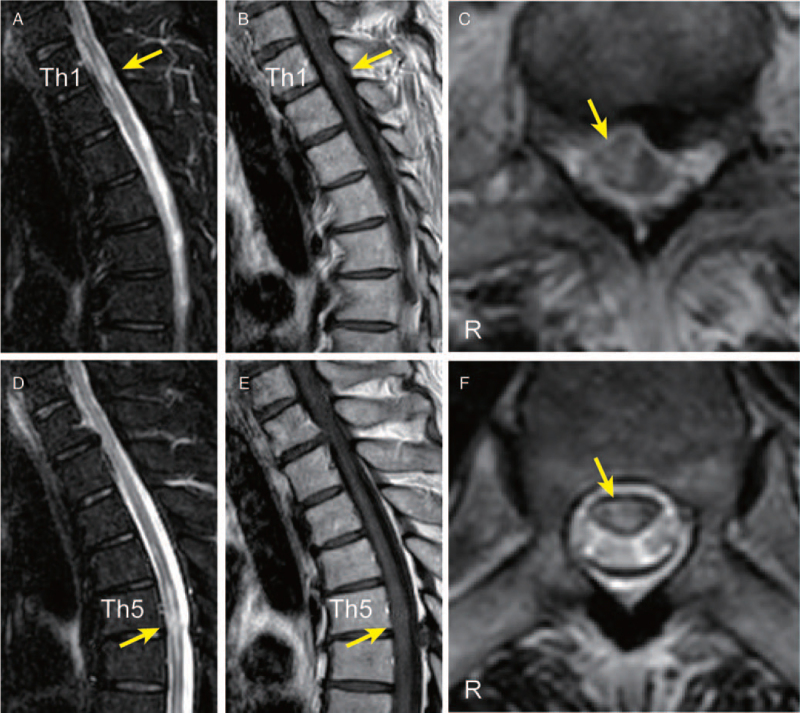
Spinal MRI. (A) Sagittal section of T2-weighed image of cervicothoracic spine shows a high-intense area located in the Th1/2 vertebral level. (B) Gadolinium-enhanced T1-weighted image shows focal weak gadolinium enhancement in the T2 high-intense area. (C) Transverse T2-weighted image shows a high-intense area in the Th1/2 vertebral level. (D–F) Other sections of the same sequence as panels A–C show another high-intense area located in the Th5/6 vertebral level.
